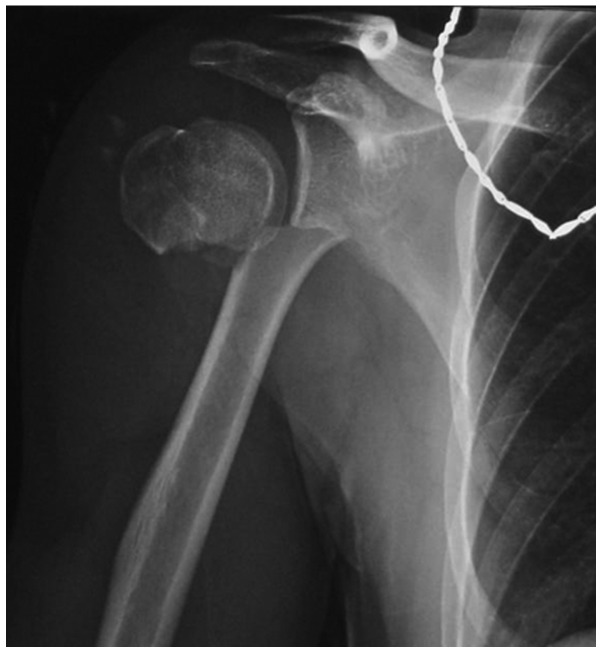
Radiograph of the right shoulder shows a fracture of the humeral surgical neck with medial displacement.
